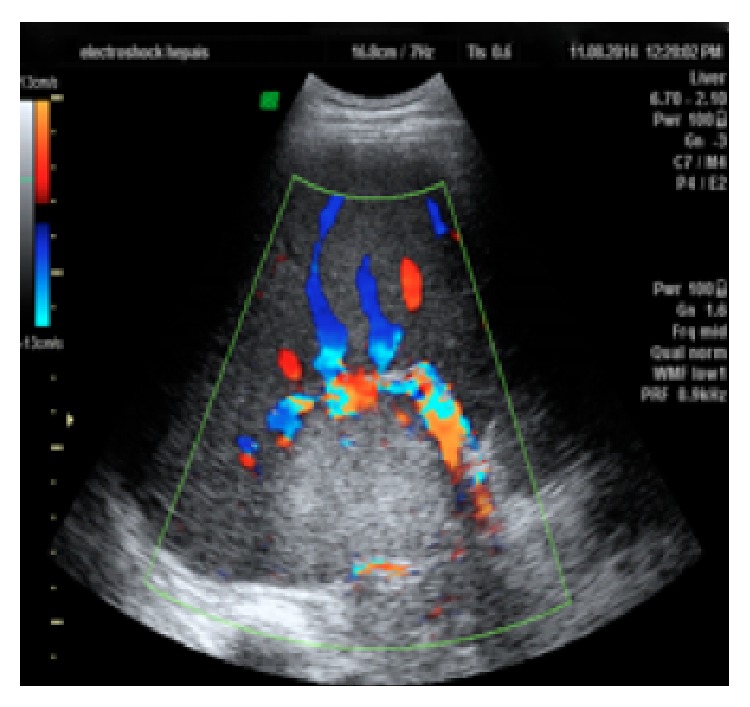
Color Doppler shows no signs of vascularization centrally, with only slight signs of vascularization around periphery.
